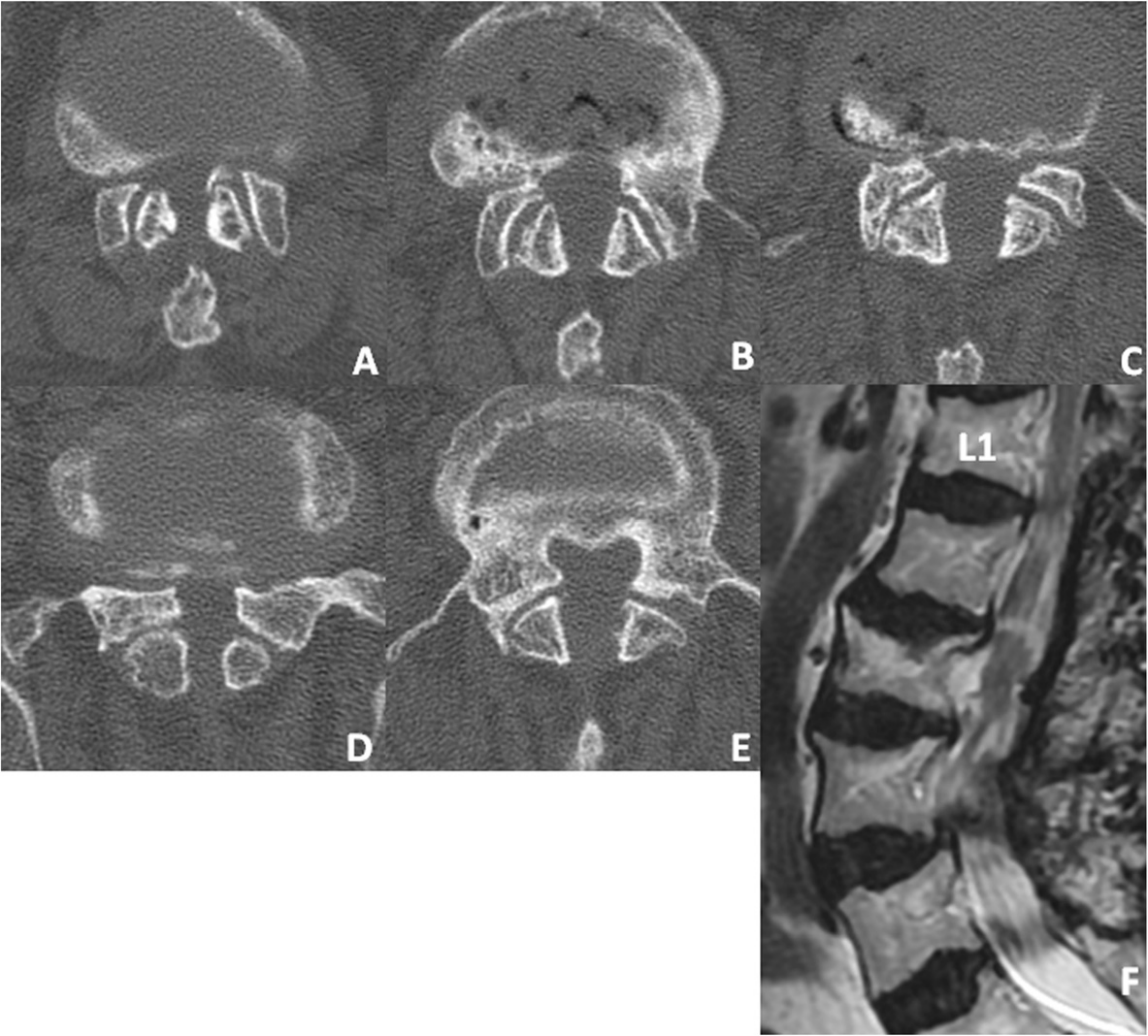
Postoperative computed tomographic and magnetic resonance images. (A) through (E) Computed tomographic scans show sufficient spinal canal enlargement at L1/2 (A), L2/3 (B), L3/4 (C), L4/5 (D) and L5/S (E) achieved by performing laminectomies with partial resection of the OLF at L1/2 and total resection of the OLF at L2/3 and L3/4. (F) Postoperative sagittal T2-weighted magnetic resonance imaging scan reveals good decompression of the dural sac from L1/2 to L4/5.
